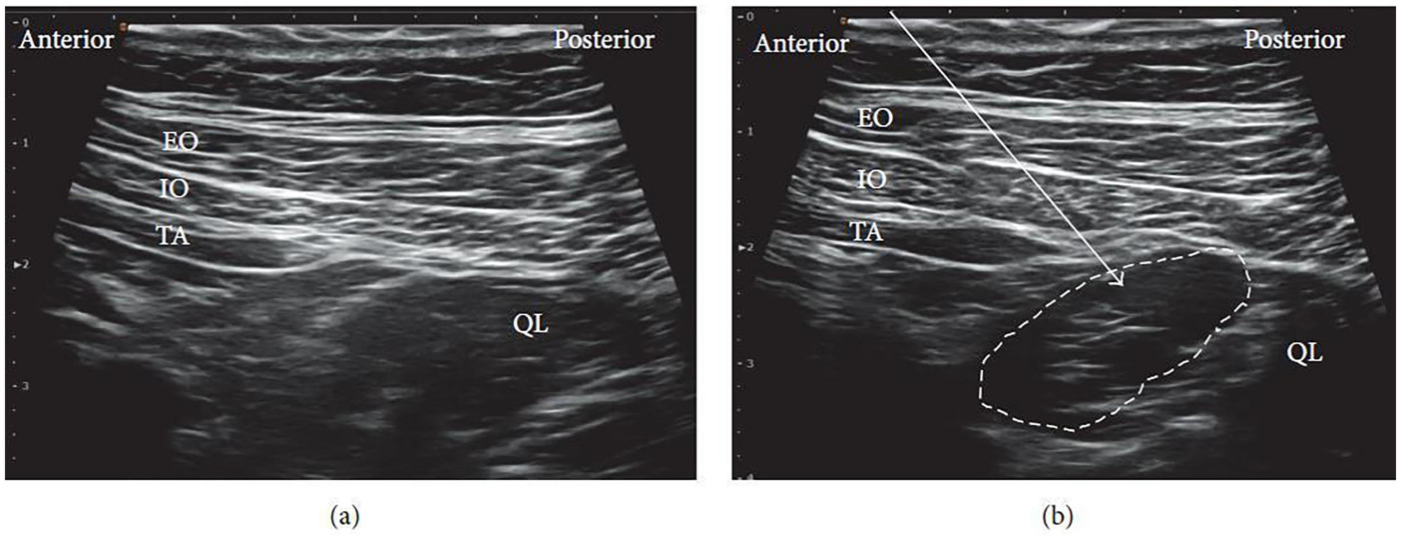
Ultrasound images of lateral QLB: (a) before injection and (b) after injection. EO, external oblique; IO, internal oblique; TA, transversus abdominis; QL, quadratus lumborum. White arrow: needle trajectory; white dotted line: spread of local anesthetic [Reproduced from Ueshima et al. (28). © John Wiley & Sons, Inc. Distributed under the Creative Commons Attribution License, CC BY 4.0].
